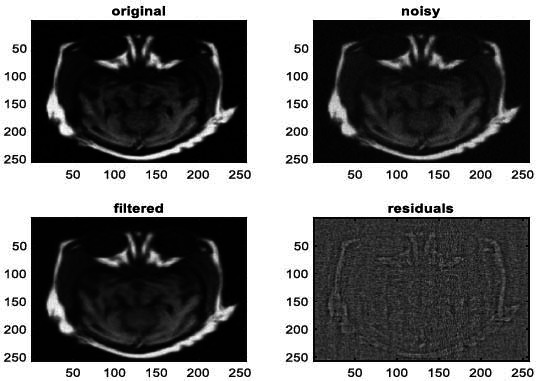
The ninth example of MRI denoising by applying the proposed image denoising technique.
